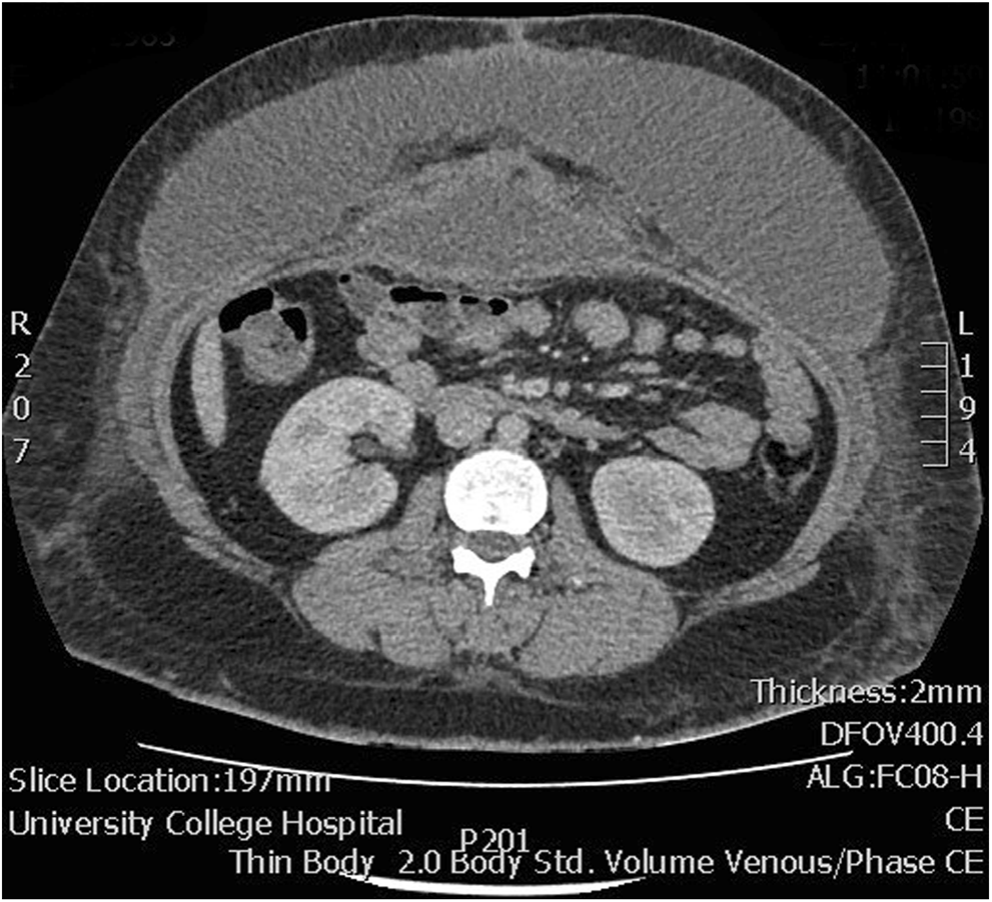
Axial CT showing a very large seroma that occurred following anterior component separation and mesh implantation
